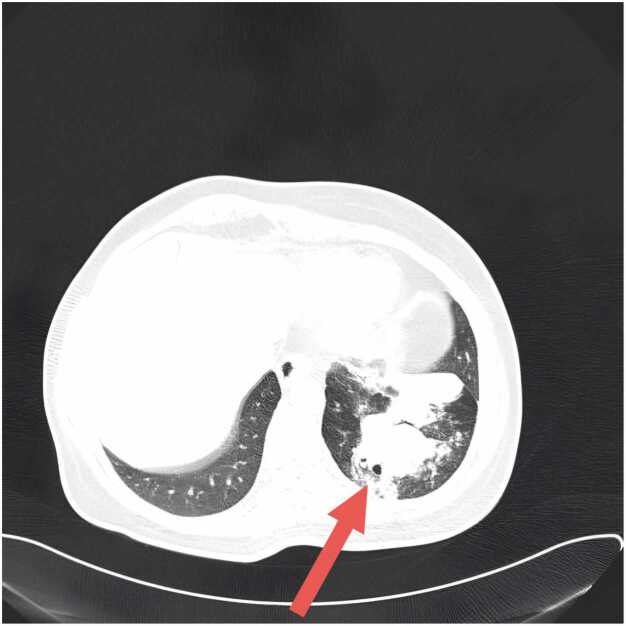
High-resolution computed tomography (HRCT) of the chest demonstrating subacute invasive pulmonary aspergillosis before treatment. A. Serial axial HRCT images (9 consecutive slices) showing a persistent area of consolidation in the left lower lobe with early cavitation. B. Single axial HRCT image demonstrating the maximal extent of the cavitary lesion in the left lower lobe. The arrow indicates areas of central hypodensity within the consolidation, representing liquefactive necrosis progressing to frank cavitation. Air bronchograms are visible within portions of the consolidated lung parenchyma. The lesion is surrounded by a rim of ground-glass opacity, characteristic of the inflammatory halo seen in invasive aspergillosis.
